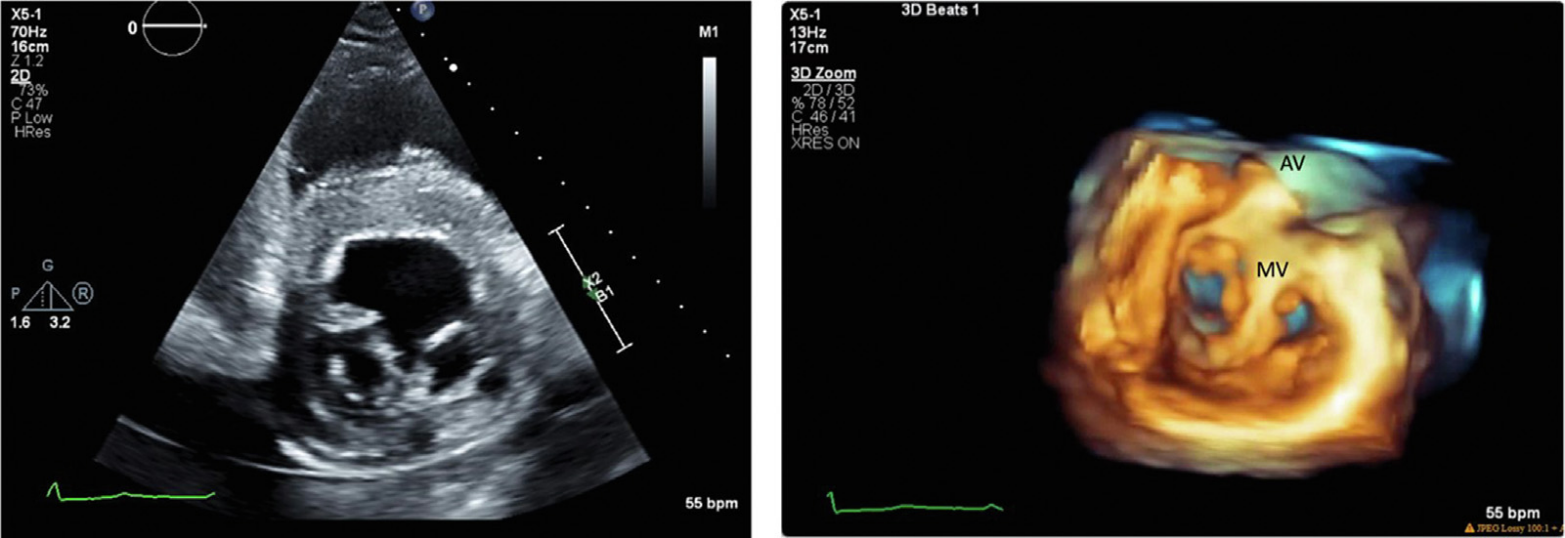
Two-dimensional TTE (*left*) and three-dimensional TTE (*right*), parasternal short-axis view at the level of the mitral annulus (diastolic phase), both showing the mitral valve at the annular level. There is a tissue bridge bisecting the center of the opening between the anterior and posterior leaflets into 2 similarly sized circular orifices. Findings are consistent with a complete bridge–type DOMV. *AV*, Aortic valve; *LV*, left ventricle; *MV*, mitral valve; *RV*, right ventricle.
